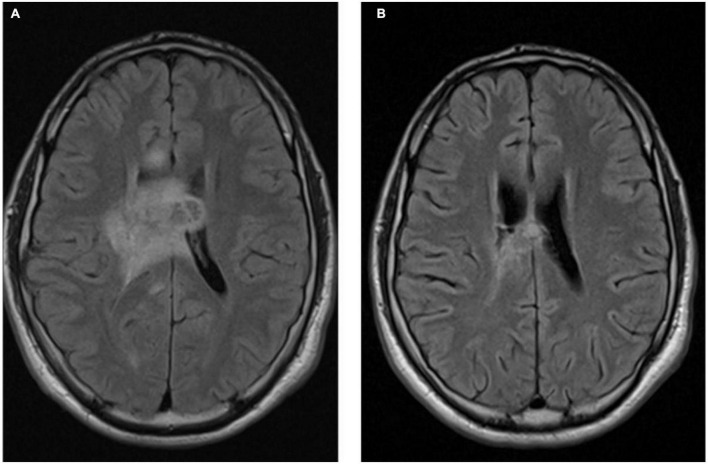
Axial T1 MRI images ganglioglioma pre-BRAF therapy (A) and 4 months post-BRAF therapy (B).
